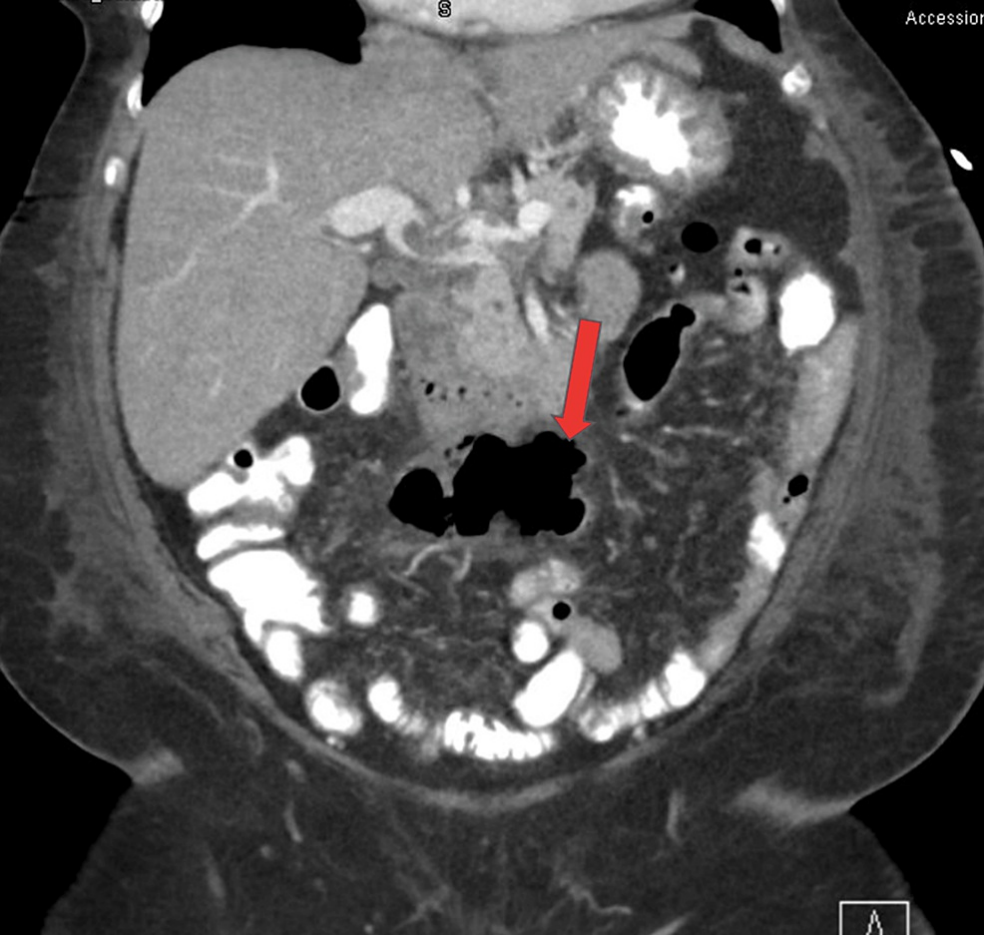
Second computed tomography of the abdomen showing enlarging mesenteric abscess extending from left to right lower quadrant
